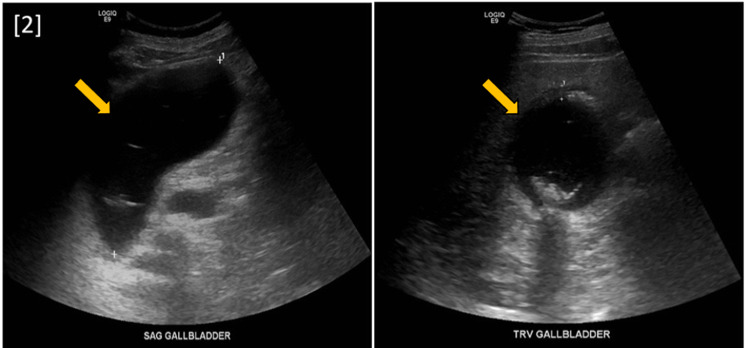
Right upper quadrant ultrasound (sagittal and transverse sections) showing moderately distended gallbladder with sludge and stones. Additionally, air present in the fundus is highly suggestive of emphysematous cholecystitis.
